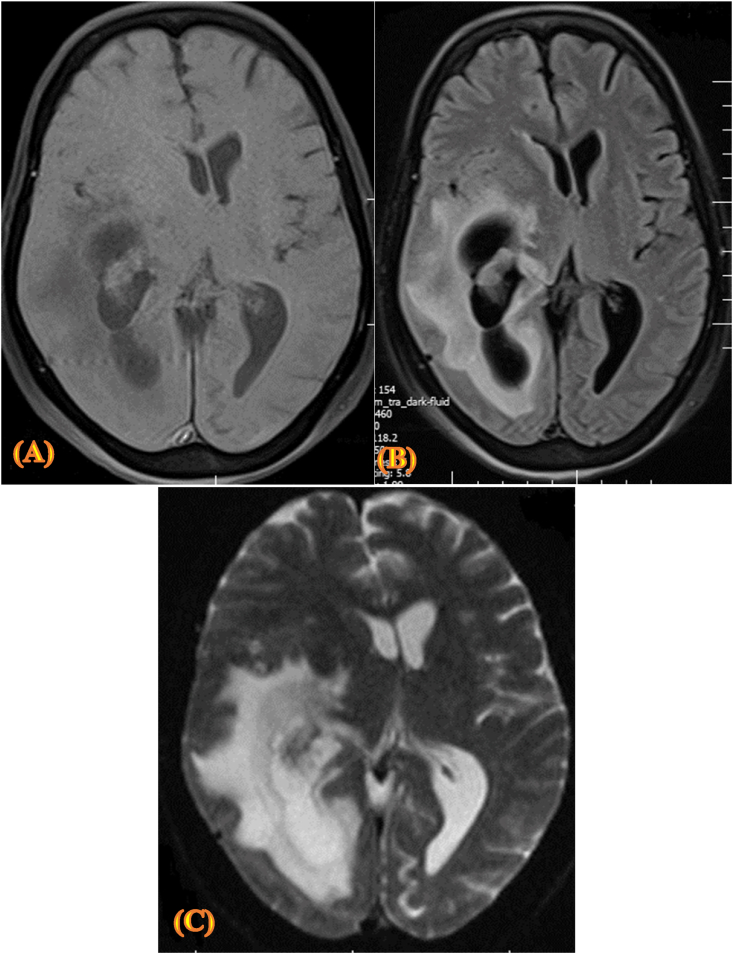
Preoperative MRI image, A; T1W, Axial view. Isointense lesion in right ventricle with midline shift. B; PD MRI image, Axial view. Perilesional edema is seen. C; T2W image. Axial view: Isointense lesion in the right ventricle with surrounding edema and midline shift. (MRI, magnetic resonance imaging; PD, proton density).
